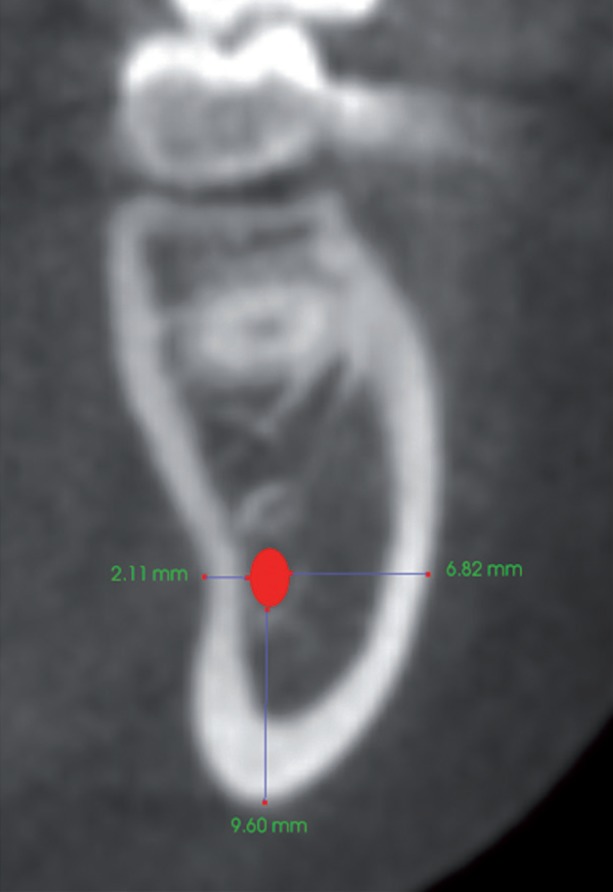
Cross-sectional CBCT image showing bone thicknesses between the mandibular canal and 3 mandibular cortical regions (inferior cortex, buccal cortex, and lingual cortex).
